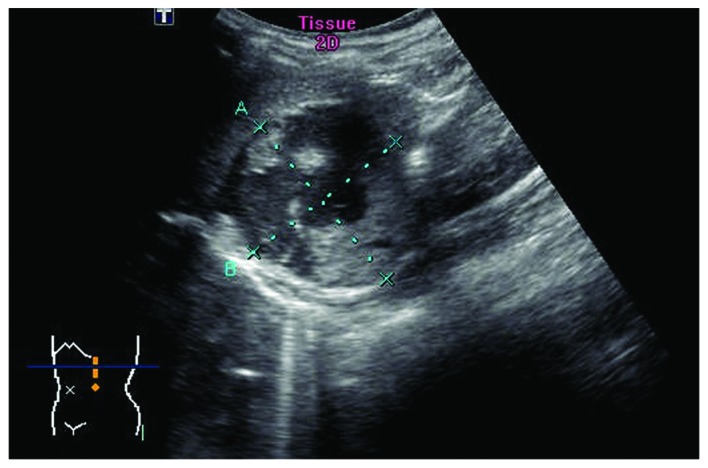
B ultrasound imaging showing a 5.9×5.3-cm retroperitoneal mass in the left adrenal region.
